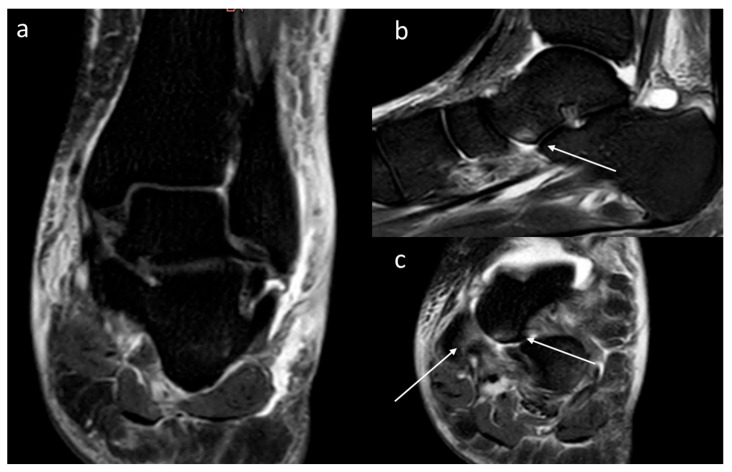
An example of bone marrow edema in an MRI examination in a patient with joint swelling, persistent pain, and allodynia on physical examination two months after a minor compressive trauma. The clinical diagnosis was CRPS in the warm phase. The MRI examination shows talo-tibial joint effusion, as well as significant thickening and diffuse edema of the subcutaneous tissue in the coronal Short Tau Inversion Recovery (STIR) sequence (a). Additionally, some focal and patchy areas of subchondral bone edema are visible at the level of the anterior portion of the talus in the sagittal (white arrows in (b)) and axial (c) STIR sequences, and at the level of the navicular bone (white arrow in (c)).
